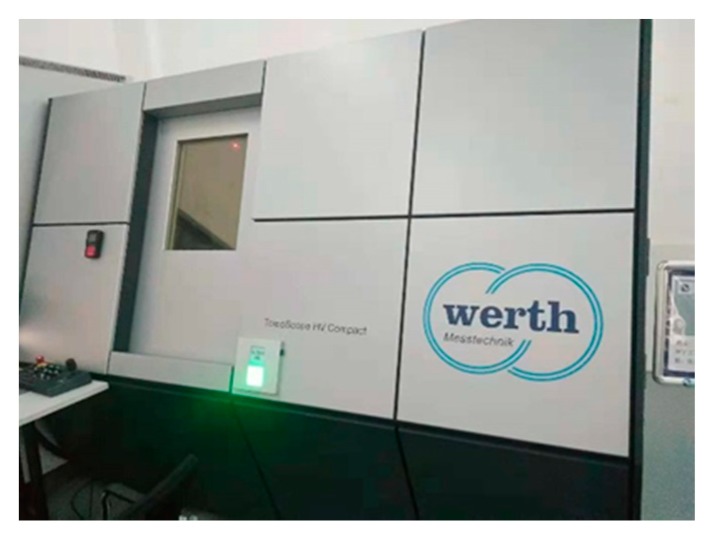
X-ray Tomography Coordinate Detector.
